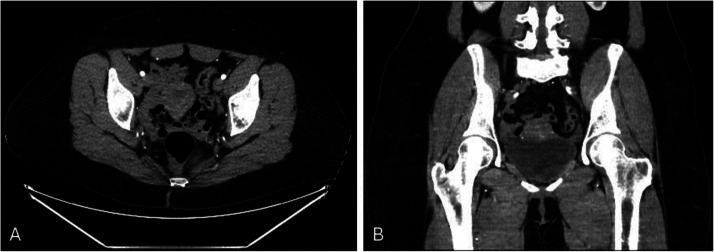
CTA images 6 weeks postembolization. As expected, when compared to CTA images shown in Fig. 3, there is no significant vascular ectasia withing the uterine parenchyma. There are no further complications such as arteriovenous fistulae or pseudoaneurysm because of the transarterial catheterization. Note: CTA, Computer tomography angiography.
